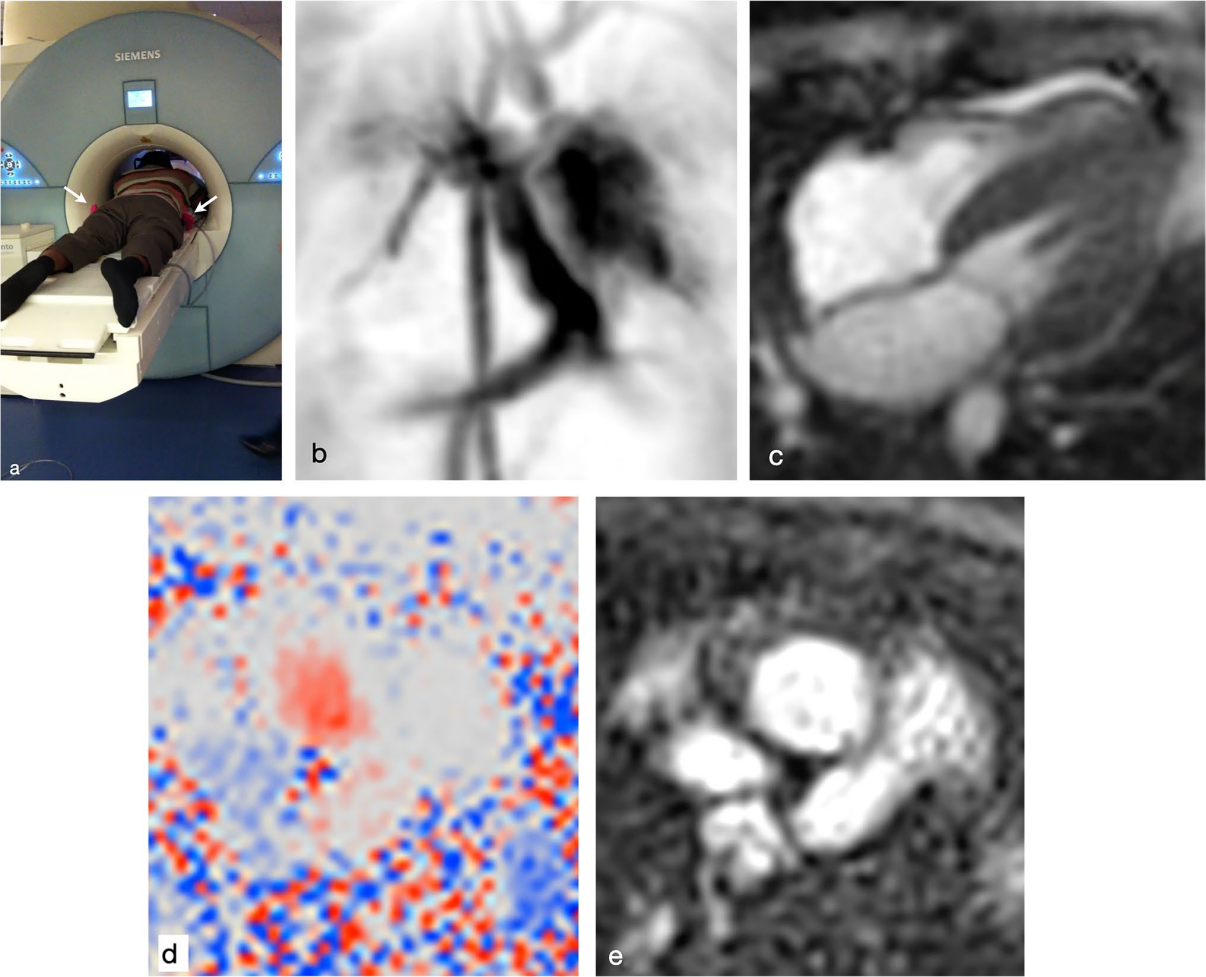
Cardiovascular MR imaging in a 3-year-old girl with pulmonary hypertension without general anaesthetic. a Clinical image shows a father comforting his child (*white arrows* show small red socks of child!) during the scan, whilst child watches a video. b Anteroposterior time-resolved gadolinium-enhanced MR angiogram of the great vessels in the thorax. c Real-time cine imaging of the heart, 4-chamber view. d, e Oblique transverse phase-contrast velocity images (phase-contrast image [d], magnitude image [e]) of the ascending aorta to measure systemic flow
